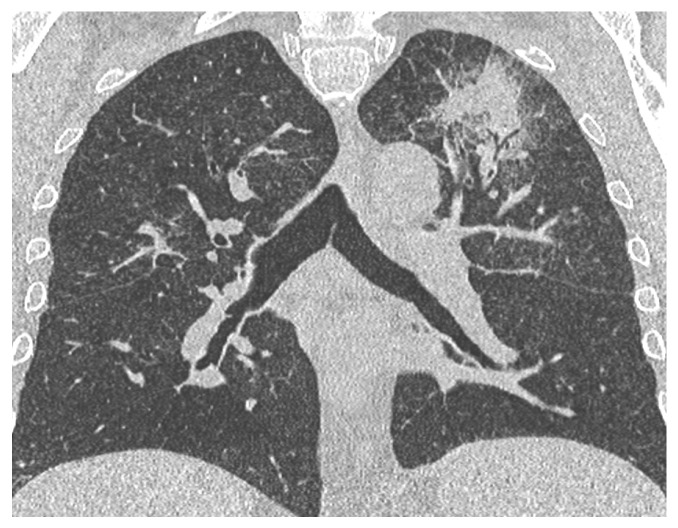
Chest CT: consolidation in the left upper lobe with bronchiectasis and positive air bronchograms, consistent with *Pseudomonas aeruginosa* pneumonia. Representative image from the anonymized institutional radiology archive of University Hospital Frankfurt.
